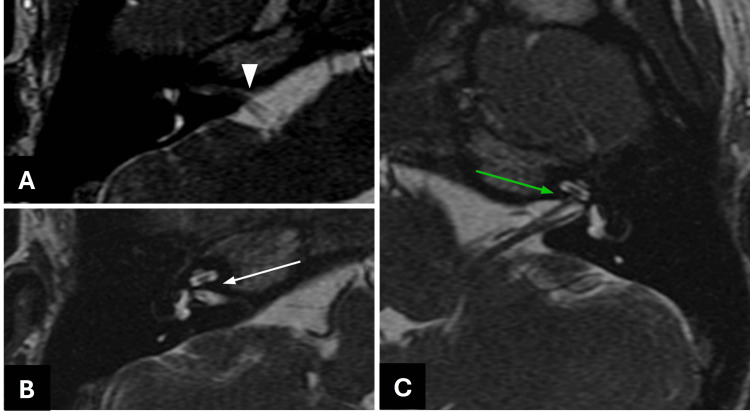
MRI temporal bone axial view on FIESTA-C sequence. (A) Facial nerve and hypoplastic vestibulocochlear nerve entering the narrow anterosuperior segment (white arrowhead) of the right-sided DIAC. (B) Preservation of right IAC fundus (white arrow) distal and inferior to the entry of the nerves is present. (C) Left cochlear nerve traversing the IAC and entering the cochlea through the cochlear aperture (green arrow) MRI: magnetic resonance imaging; IAC: internal auditory canal; DIAC: duplicated internal auditory canal; FIESTA-C: fast imaging employing steady-state acquisition with contrast
